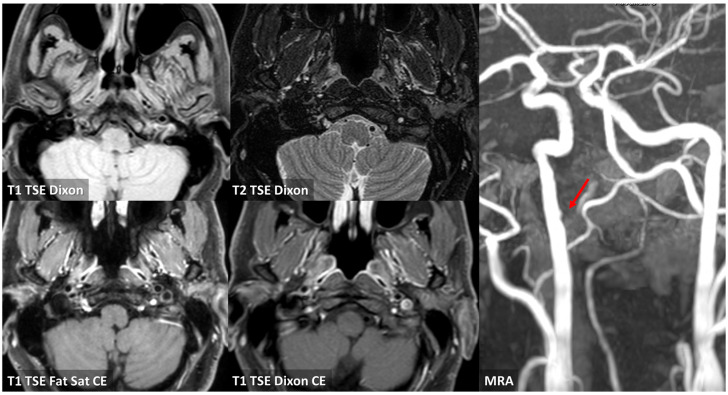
MRI with vessel wall imaging and MRA 4 months after ischemic stroke showing an almost complete healing of vessel damage in the prepetrous segment of the right ICA with only a small eccentric thickening of the posterior wall, hyperintense in T1 and T2 Dixon sequences with a peripheral contrast enhancement. The corresponding MRA shows a very mild irregularity in the luminal profile of the right ICA in the prepetrous segment (red arrow).
